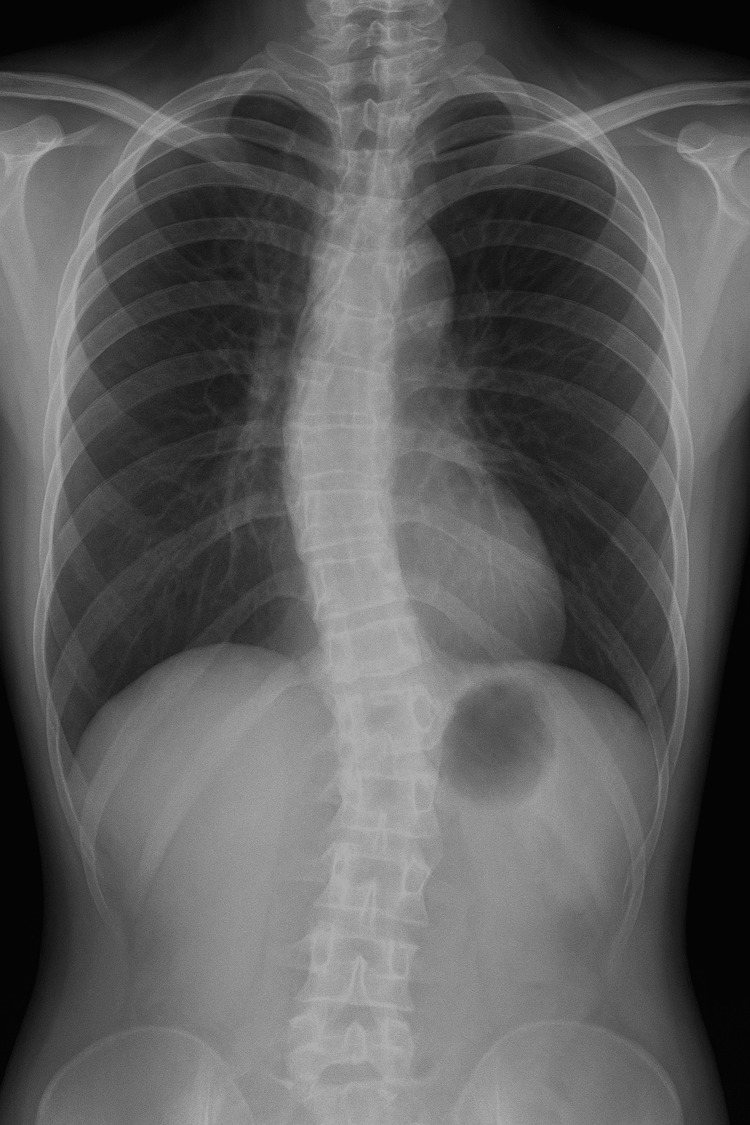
X-ray showing S-shaped scoliosis
